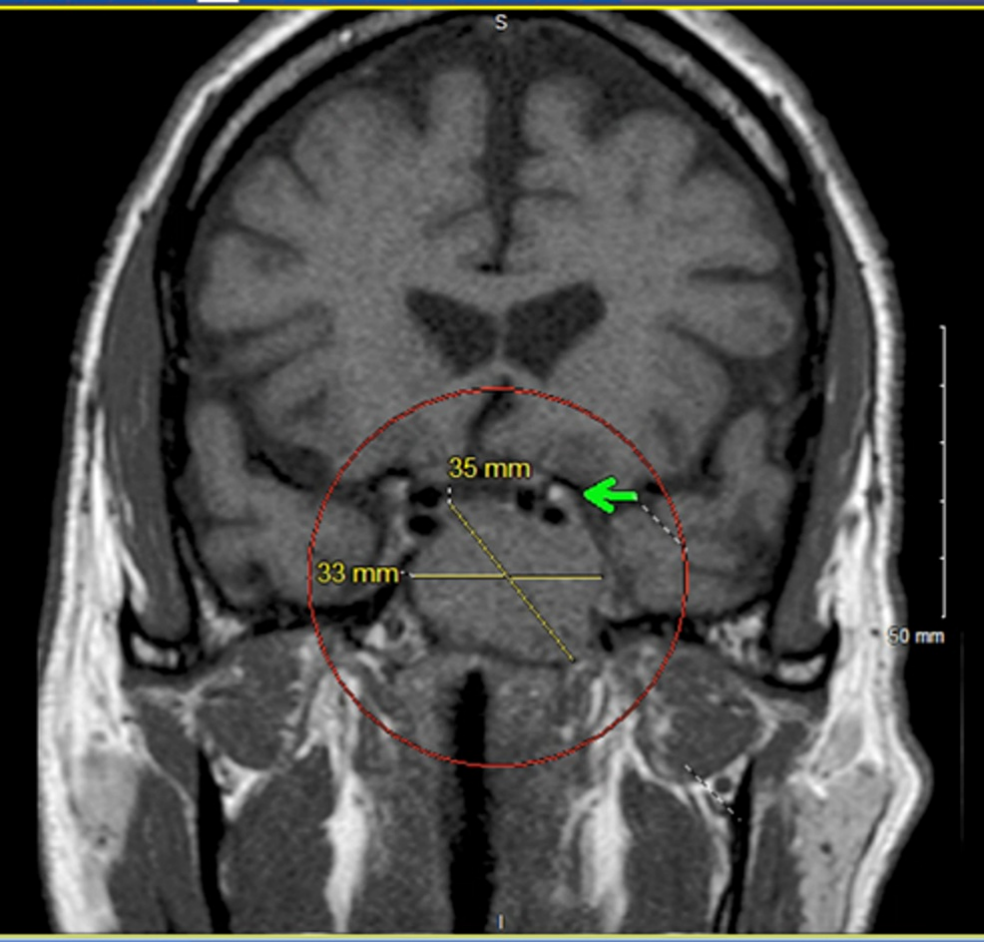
Coronal T1-weighted MRI sequence showing hypointense 35 mm × 33 mm clivus mass extending to partially encase cavernous segment of the internal carotid artery (green arrow). MRI: magnetic resonance imaging
